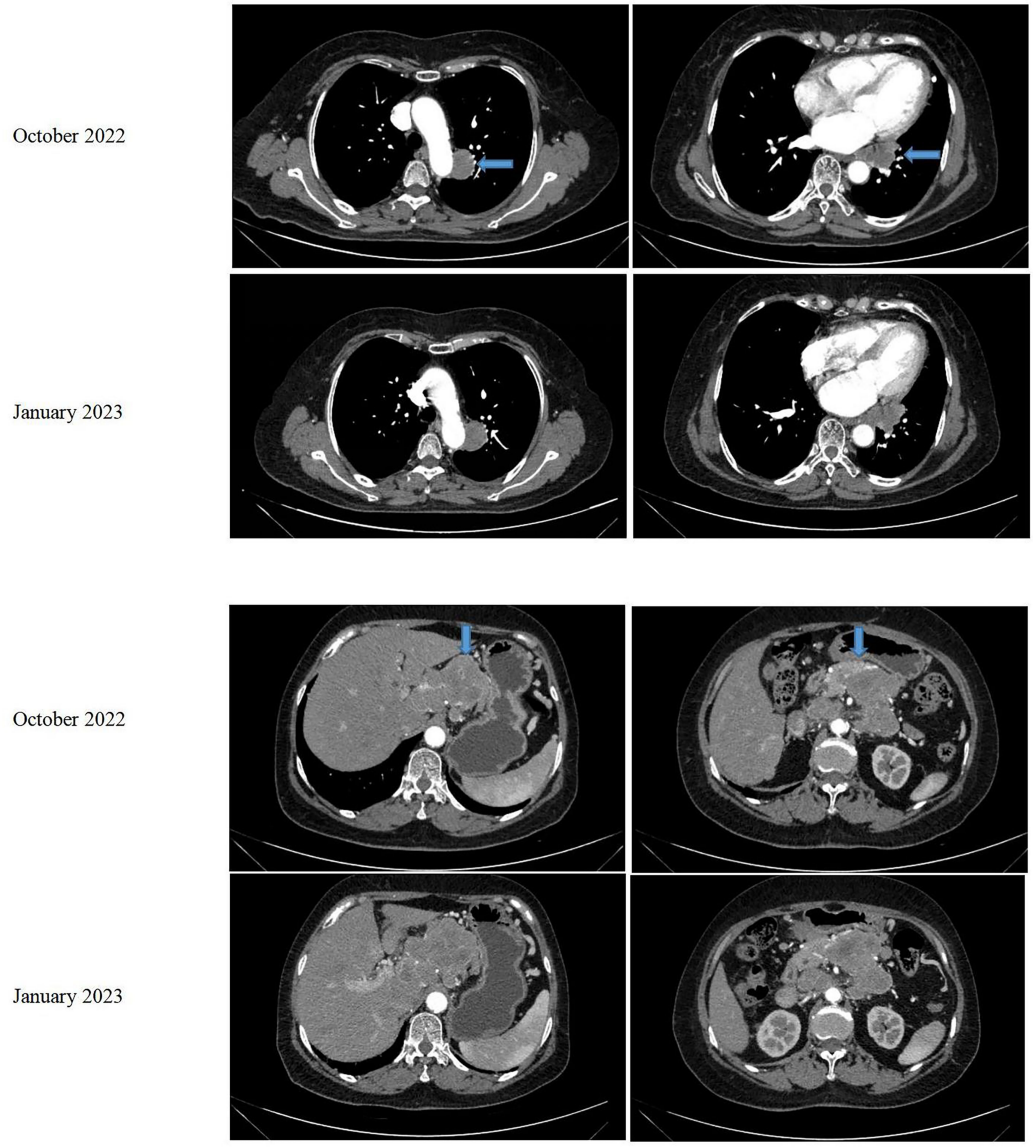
CT of the patient’s chest and abdomen in October 2022 and January 2023.
